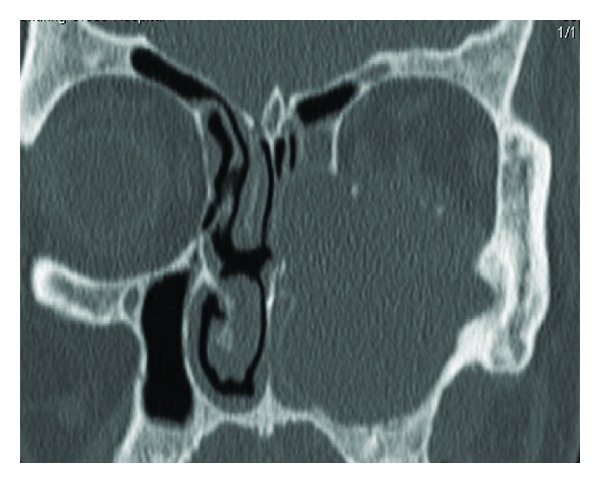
A coronal CT scan of a left maxillary mucocele eroding through the orbital floor and medial antral wall.
